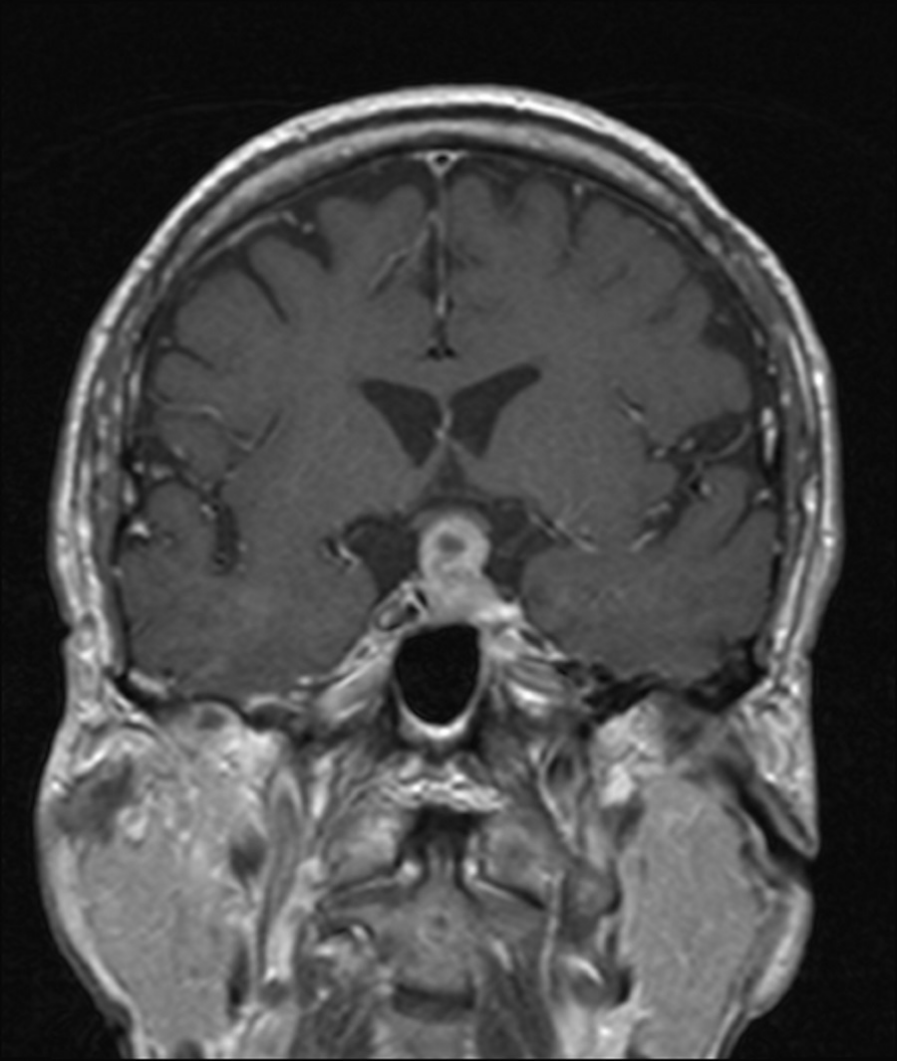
Initial MRI obtained at the time of diagnosis Initial T1 coronal MRI showing a uniformly enhancing sellar mass extending into the suprasellar cistern with mass effect on the optic chiasm.
